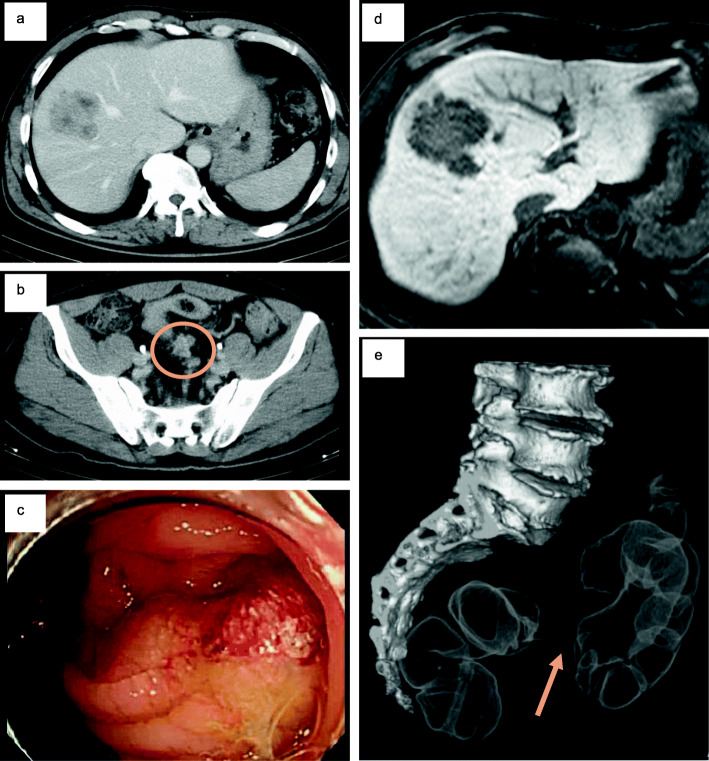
Pretreatment computed tomography (CT), magnetic resonance imaging (MRI), and colonoscopy. a Axial view of a liver metastasis on contrast-enhanced CT. b Axial view of regional lymph node metastases (circle) on CT. c Primary sigmoid colon cancer on colonoscopy. d Axial view of a liver metastasis on ethoxybenzyl diethylenetriamine-enhanced MRI. e Primary sigmoid colon cancer on CT-colonoscopy
